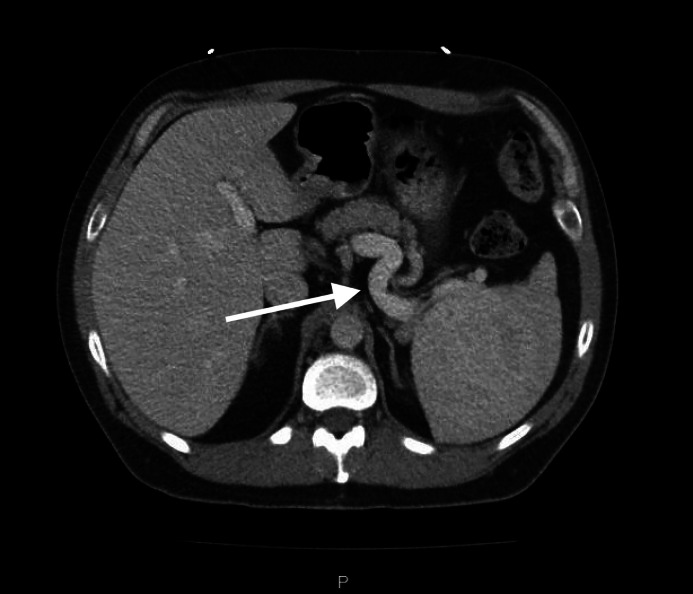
Axial view CT w/ contrast demonstrating enlarged splenic vein.
